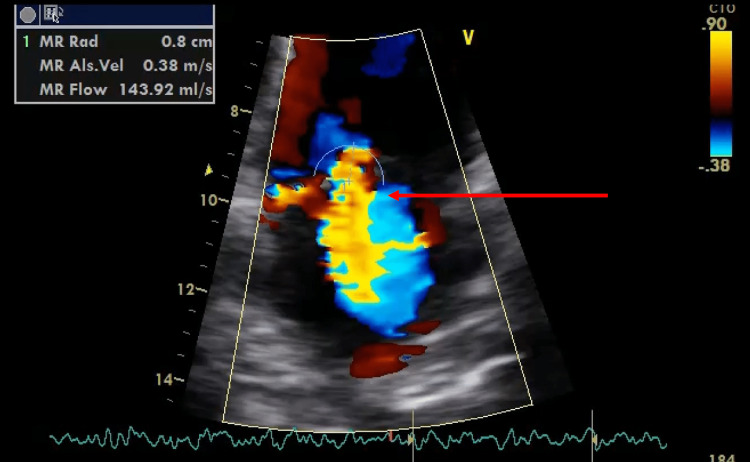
Transthoracic echocardiogram with colour Doppler showing mitral regurgitation.
